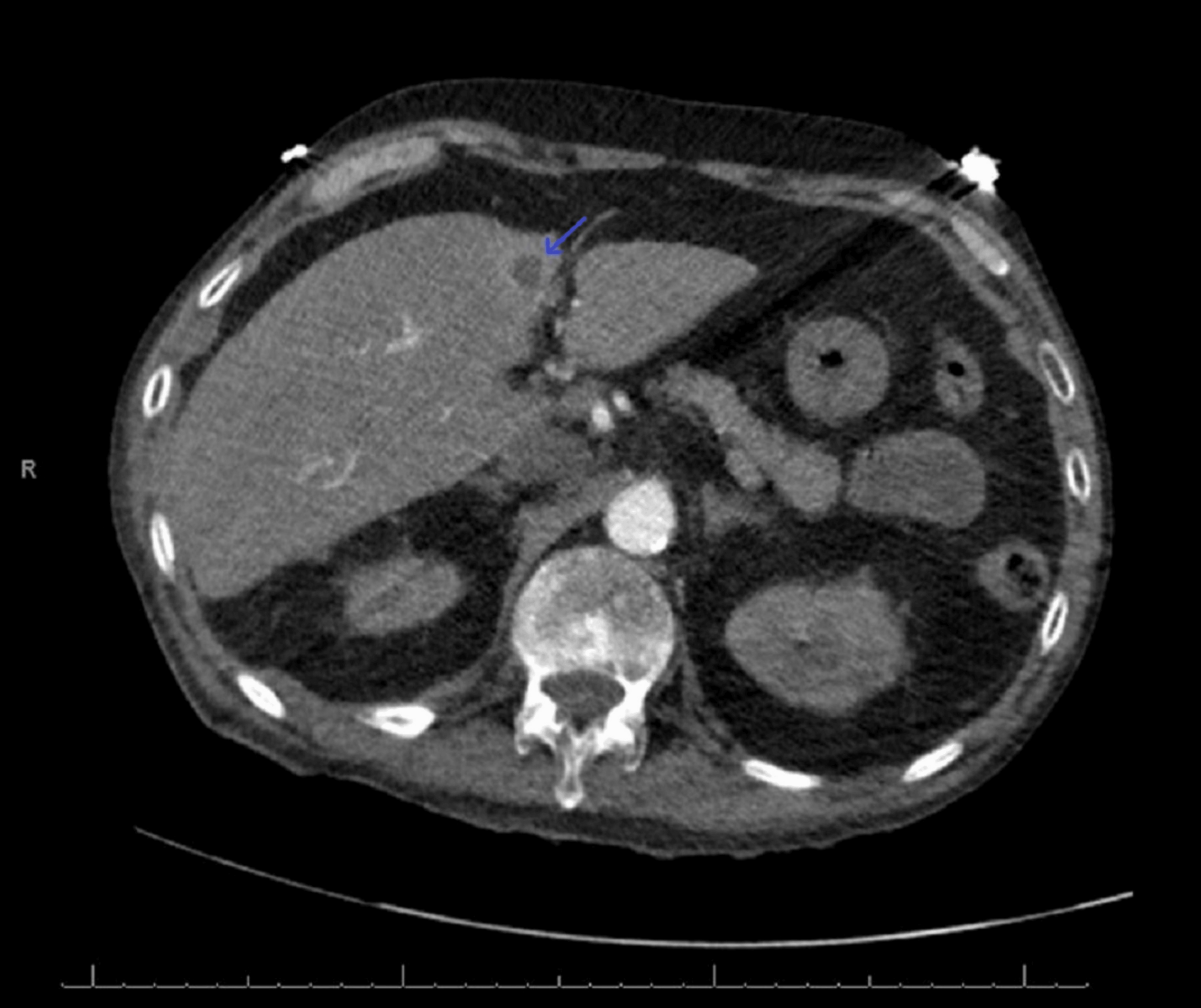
CTA chest with contrast Arrow: metastasis in the liver CTA: computed tomography angiography
